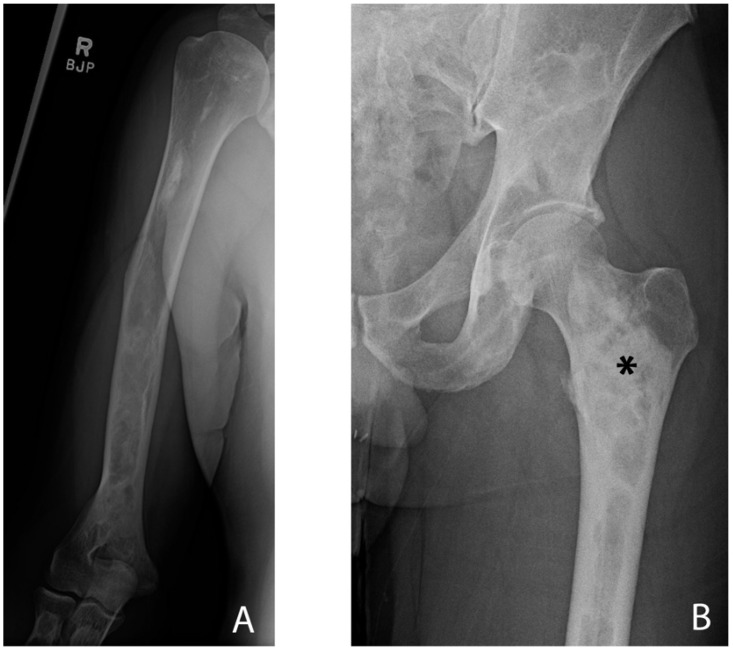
Radiographs of a patient with fibrous dysplasia. (A) The humerus exhibits a wide medullary canal with endosteal resorption and very thin cortices. Heterogeneous medullary patterns indicate fibro-osseous matrix with prominent lytic areas. (B) The proximal femur displays faint compressive trabeculae in the femoral head and neck and replacement of the trabecular bone. Replacement of the medullary canal with fibro-osseous matrix can be observed, especially in the inter- and sub-trochanteric areas (asterisk). Extensive endosteal resorption is apparent. Images displayed with patient consent.
